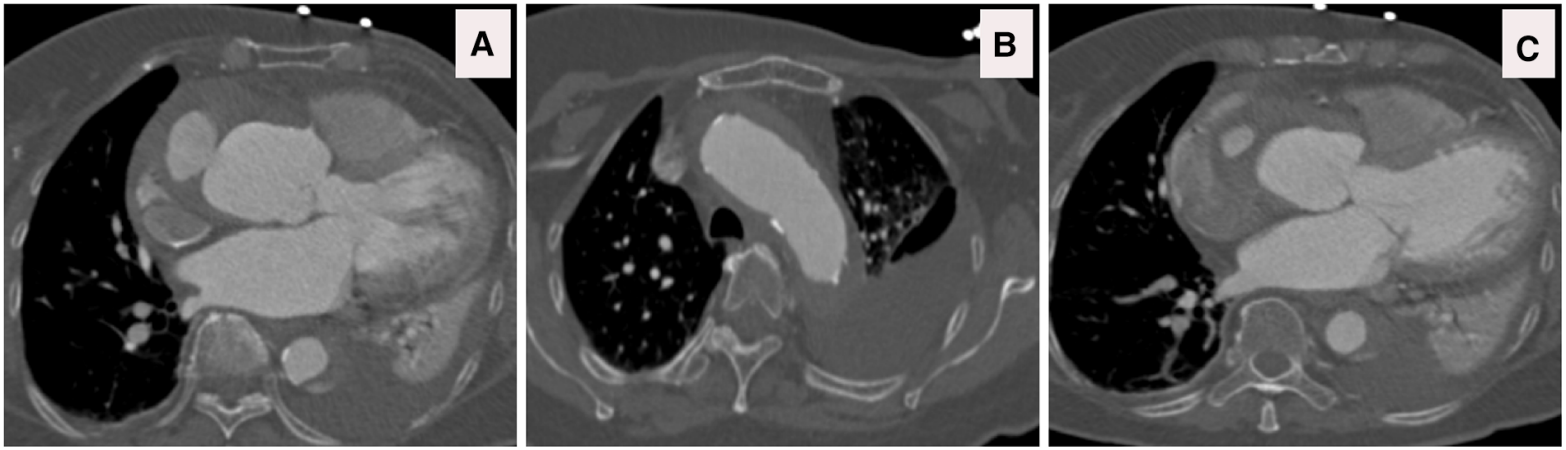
(A–C) CTA axial views demonstrated rupture of the ascending and descending aorta with acute catastrophic haemorrhage with a terminal event on patient arrival to our institute.
